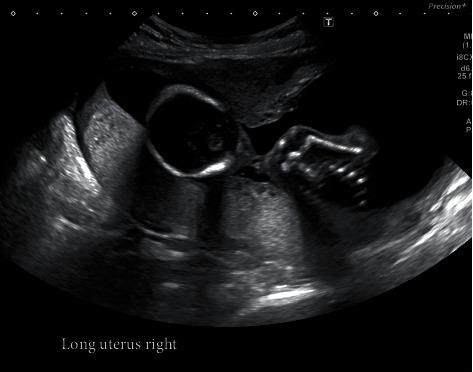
This transabdominal ultrasound image shows a right parasagittal plane at 18 weeks of gestation during the first pregnancy. A posterior placenta can be seen. Part of the uterus which appears to be a portion of the septum is seen anteriorly abutting the fetal head.
